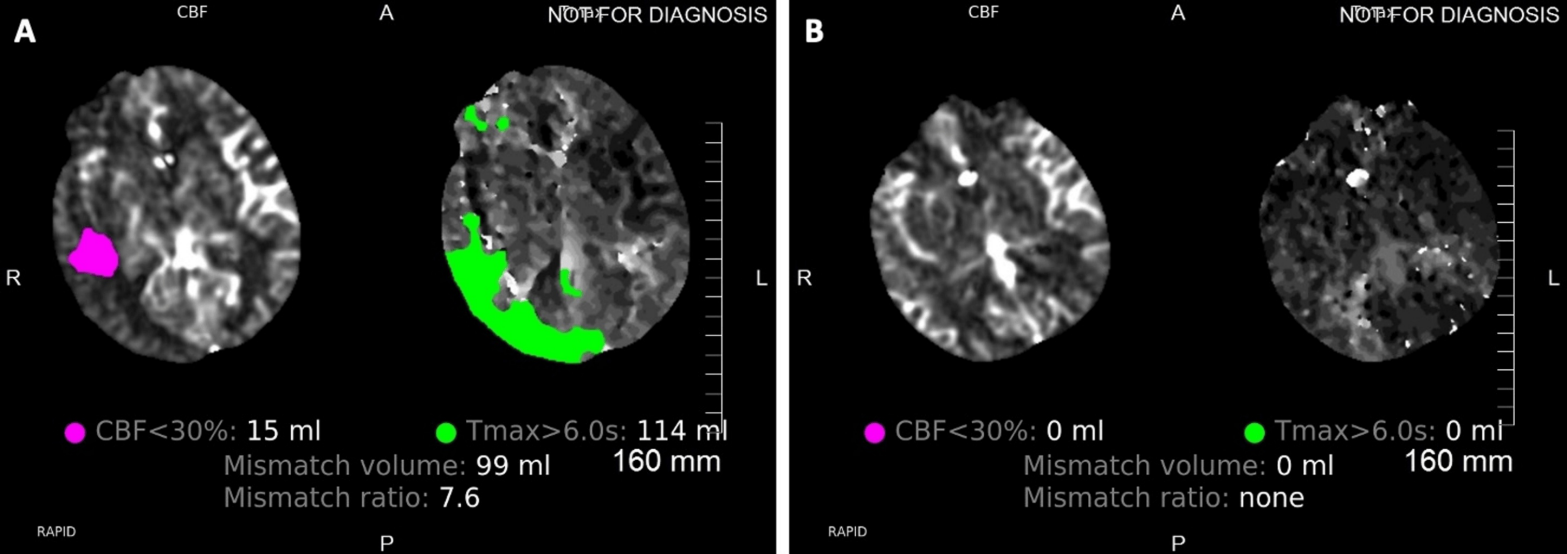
CTP demonstrating perfusion deficits during vasospasm (A) and follow-up CTP with resolved perfusion (B) deficits two days after initiation of cilostazol and albumin treatment in patient 1 CTP: computed tomographic perfusion
